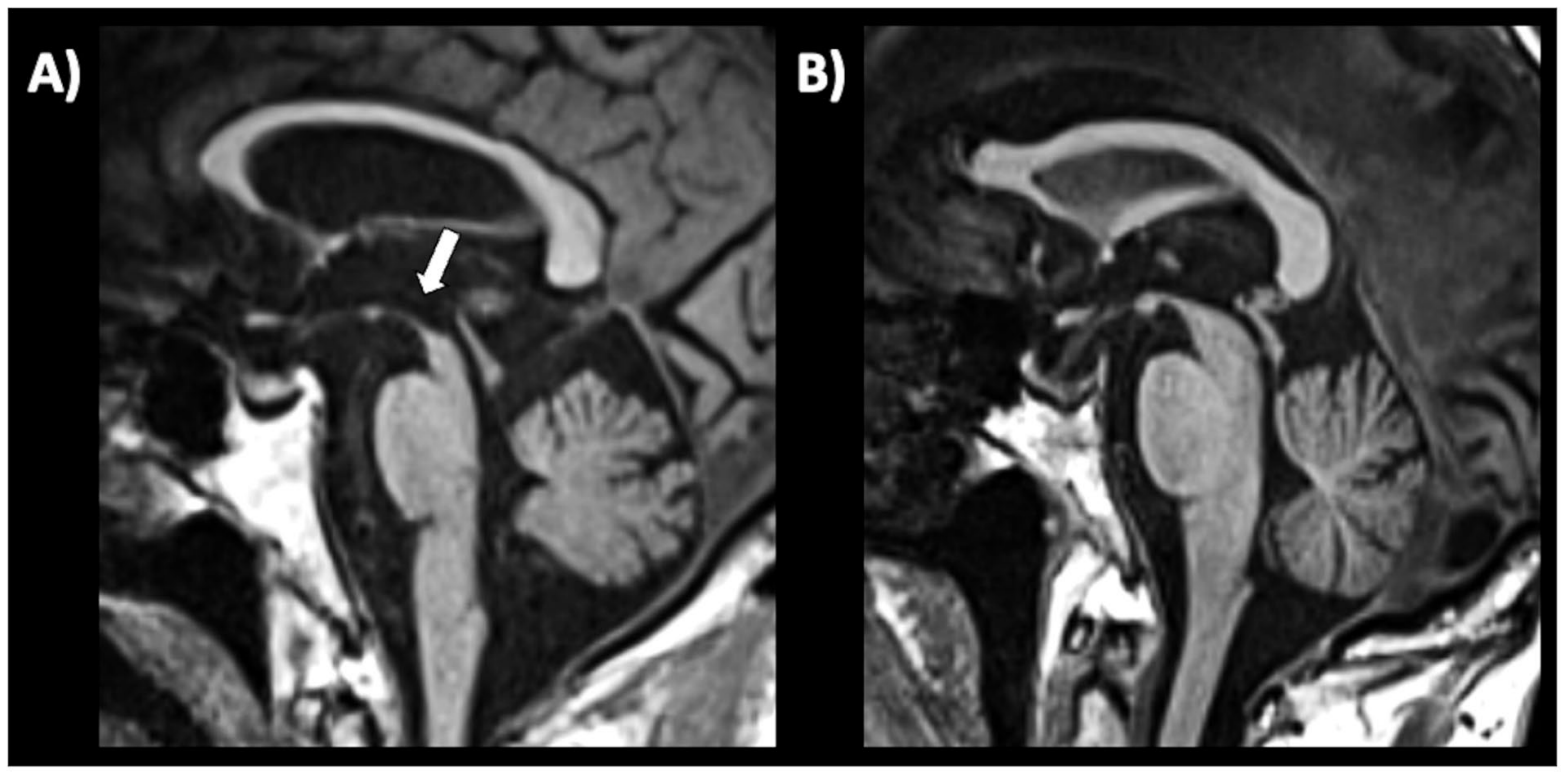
A 3T brain T1-weighted midsagittal MR image showing midbrain atrophy with hummingbird sign in a patient with progressive supranuclear palsy (A) and the normal midbrain appearance in a patient with Parkinson’s disease (B). A concave midbrain tegmentum is visible in the image on the left, forming the “beak” of the hummingbird.
